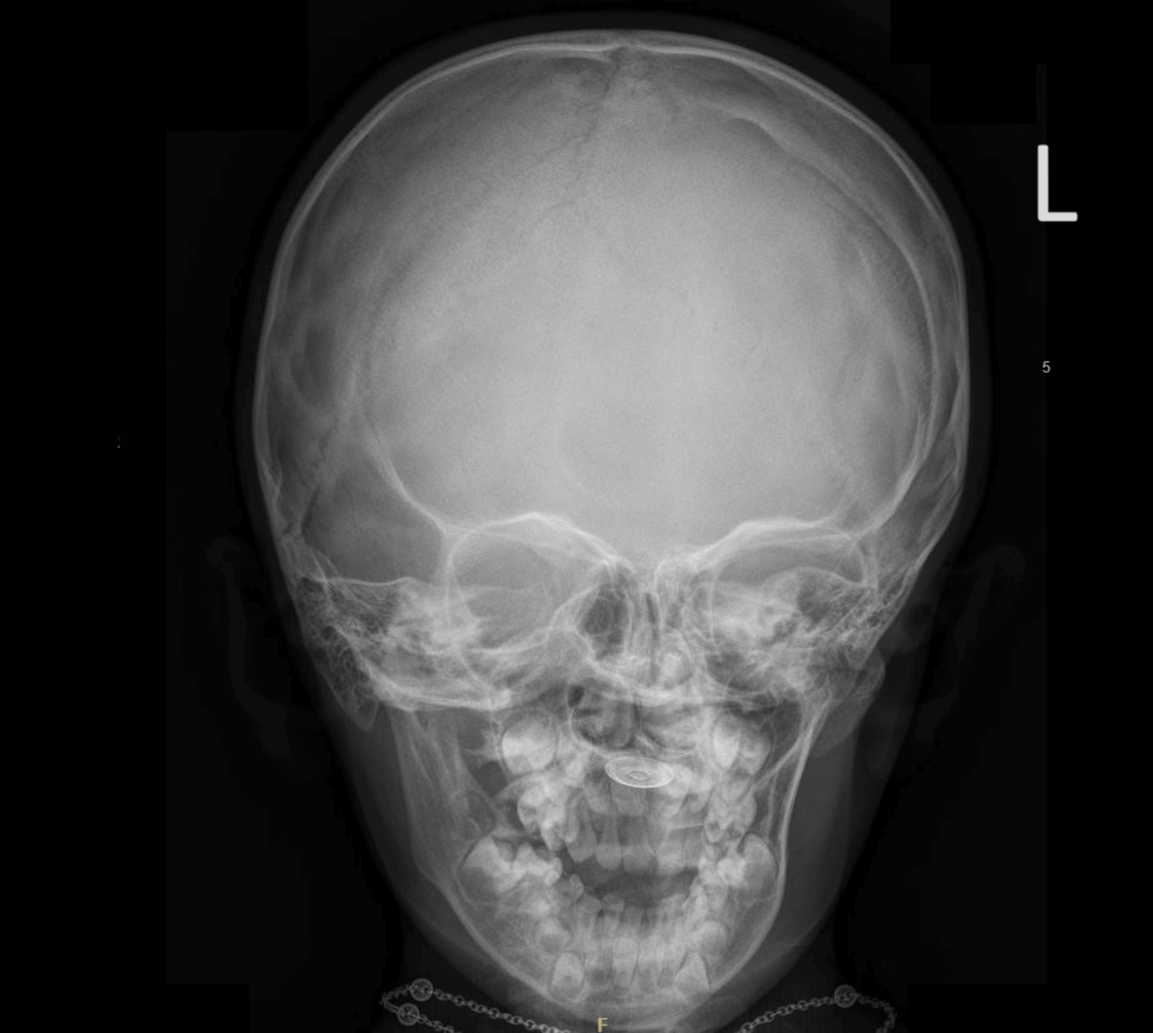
X-ray showing a round foreign body in the hard palate. Anteroposterior view skull X-ray showing a flat and round foreign body in the hard palate.
